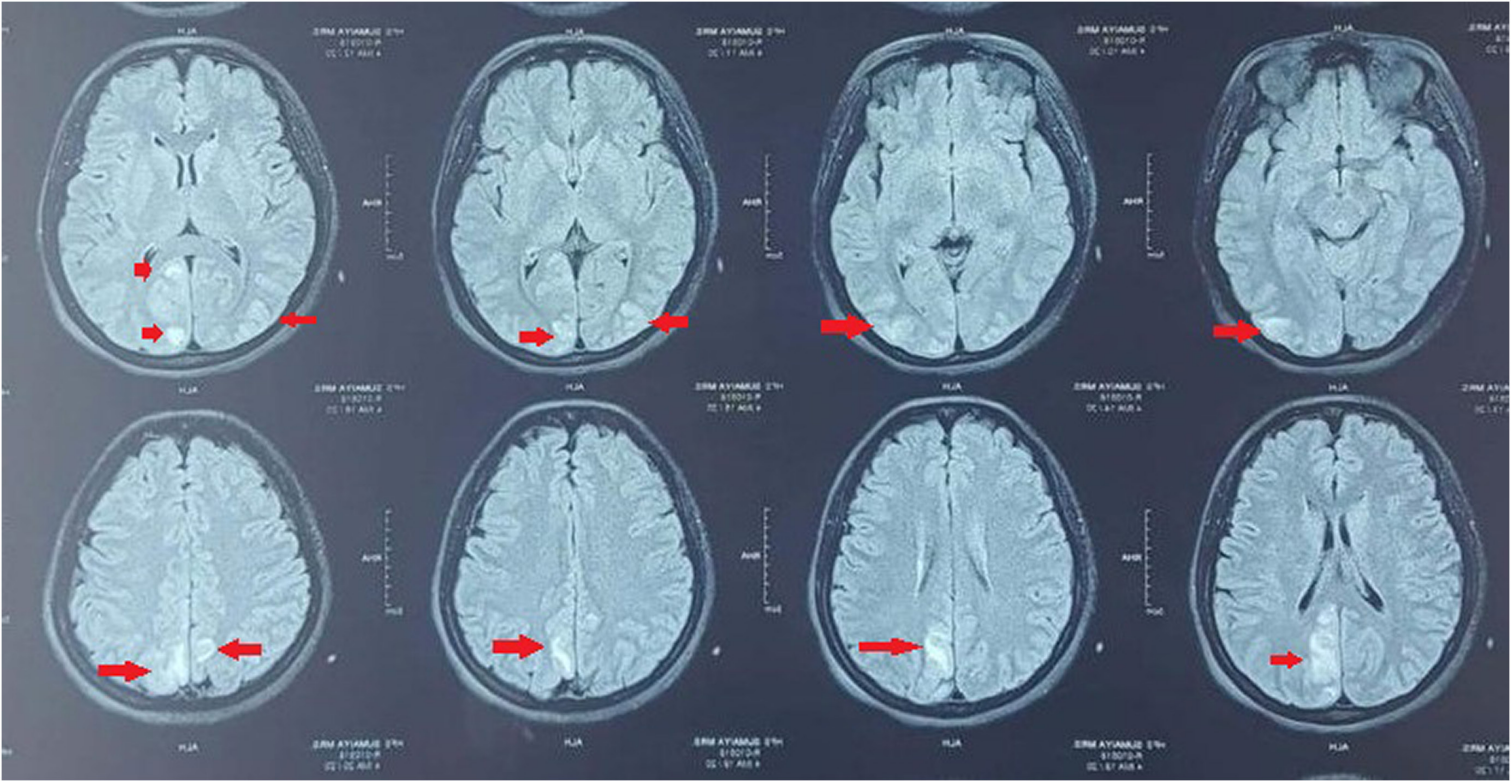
T2‐FLAIR hyperintense areas with mild restricted diffusion are seen in both posterior parietal and occipital regions. Similar signal areas are also seen in posterior‐inferior aspects of cerebellum.
